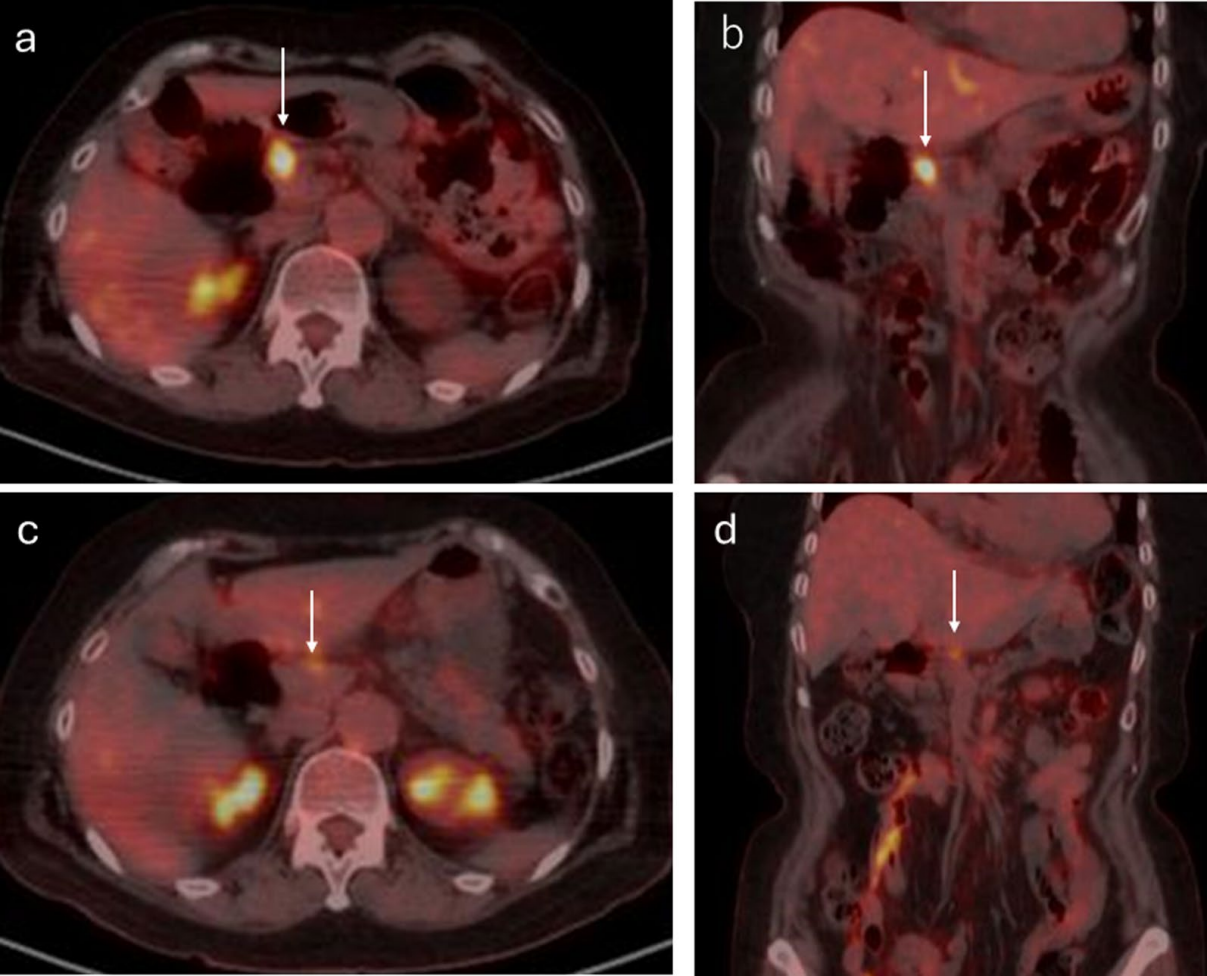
Fluorodeoxyglucose-positron emission tomography (FDG-PET) findings. a, b Before neoadjuvant chemotherapy, FDG-PET showed a standardized uptake maximum value of 6.6 in the pancreatic head. c, d After chemotherapy, FDG-PET showed a decreased accumulation with a standardized uptake maximum of 3.6 in the tumor
